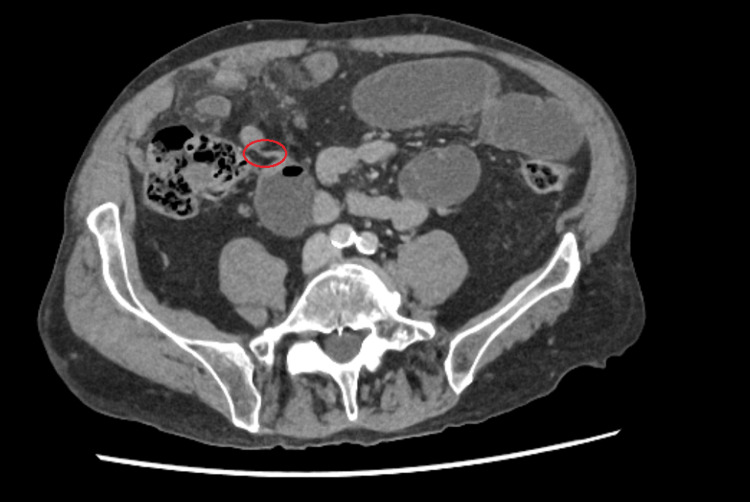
Portal venous phase CT abdomen – axial view demonstrating small bowel obstruction with jejunal and ileal dilation and the transition point
